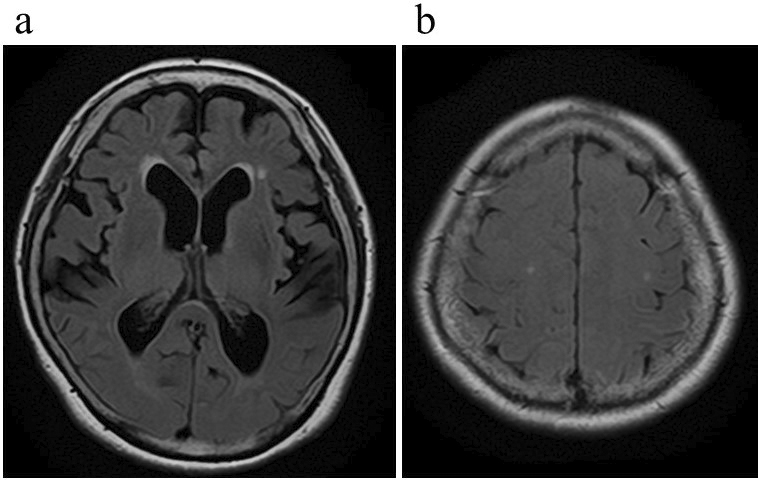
Magnetic resonance imaging showed disproportionately enlarged subarachnoid space hydrocephalus, such as ventriculomegaly with an Evans’ index of 0.35 (a), narrowing of the cerebrospinal fluid spaces near the vertex, and widening of the Sylvian fissure (b).
